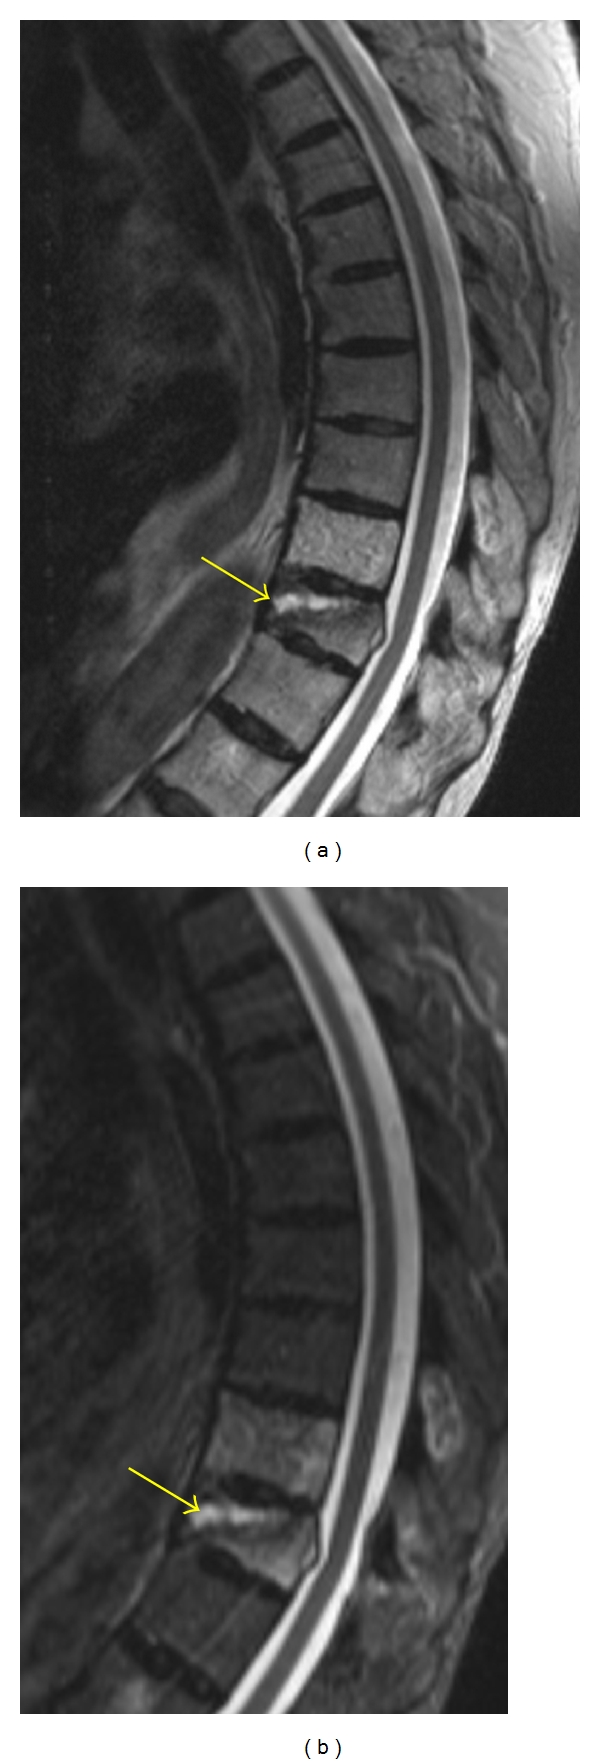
Sagittal T2-weighted (a) and STIR (b) MR images reveal abnormal hyperintense signal in a lower thoracic vertebral body due to fracture-related edema. A “fluid sign” is demonstrated in this vertebral body (yellow arrow), which is characteristic of a benign osteoporotic fracture.
